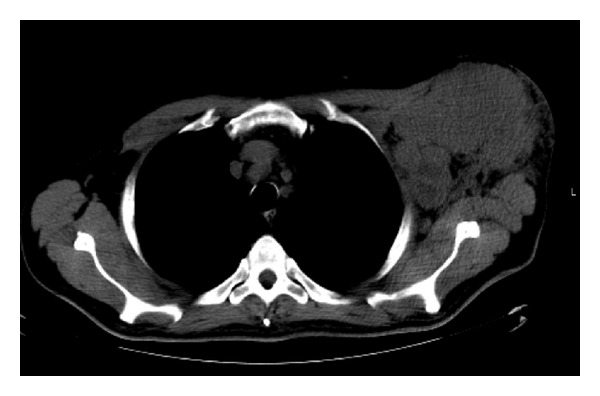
CT scan demonstrating an enlarged lymph node in left axilla with hypodense areas.
